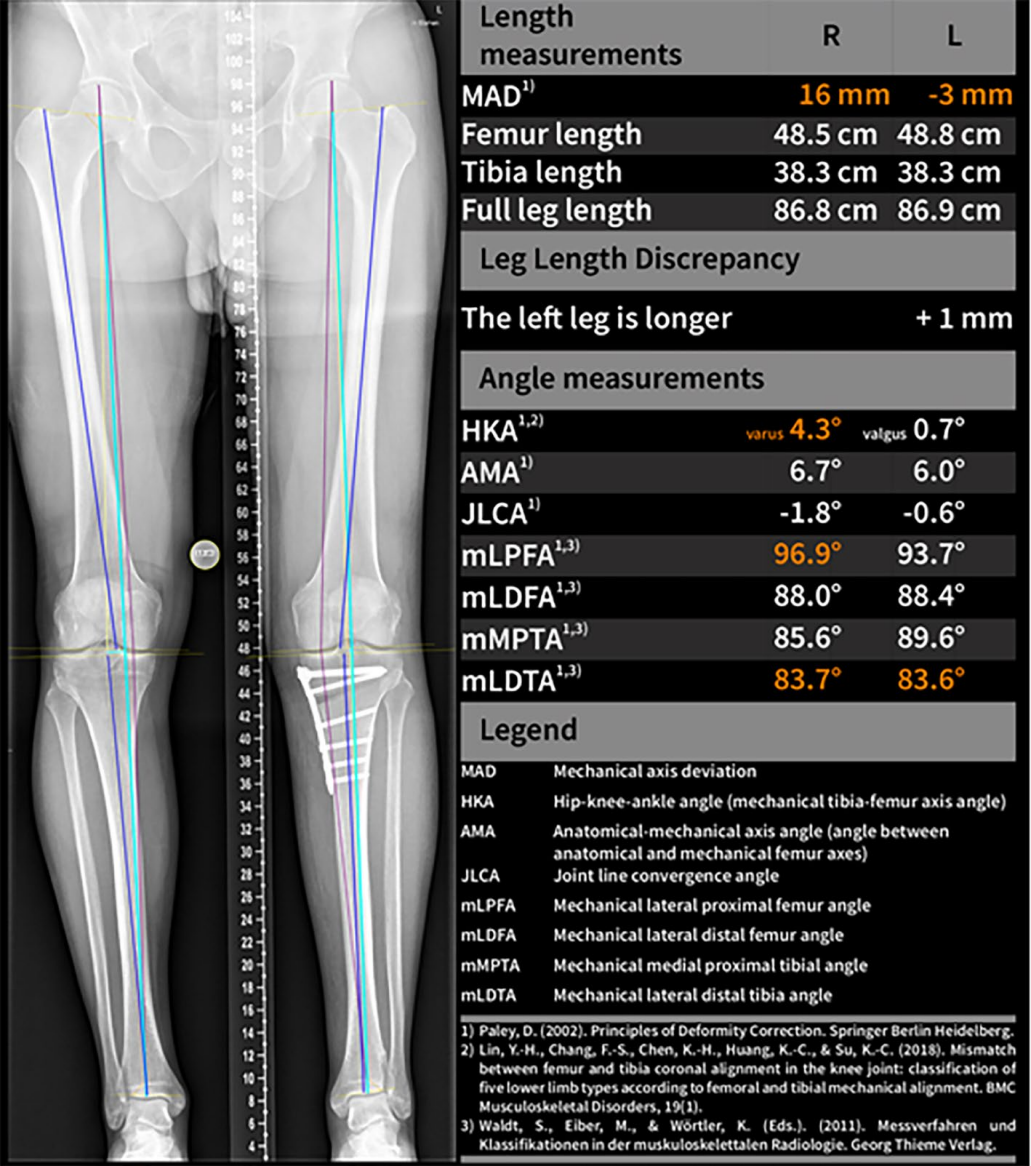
AI software (LAMA™) report of a bilateral weight-bearing long-leg radiograph after medial opening wedge osteotomy providing fully automated measurements
